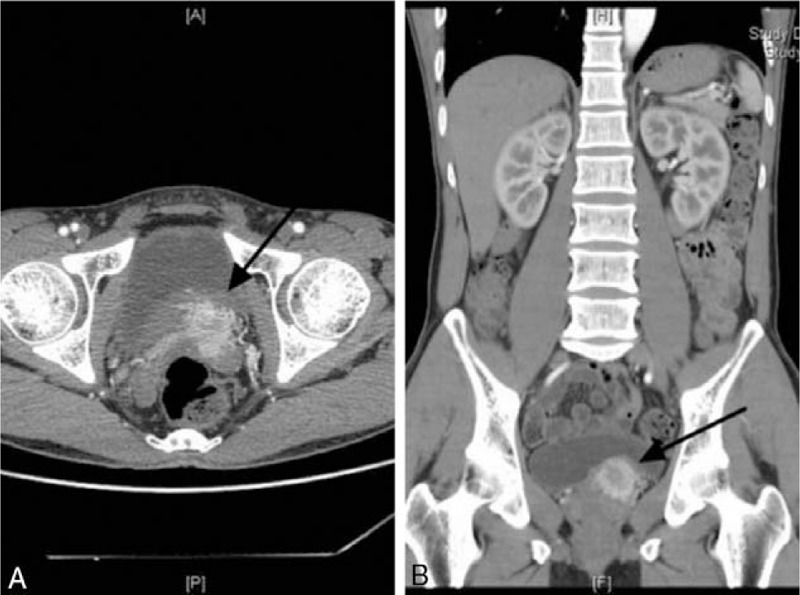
Contrast enhanced computed tomography (CT): Transverse (A) and frontal (B) views reveal a 29 mm nodular lesion on the left prostate (arrows).
